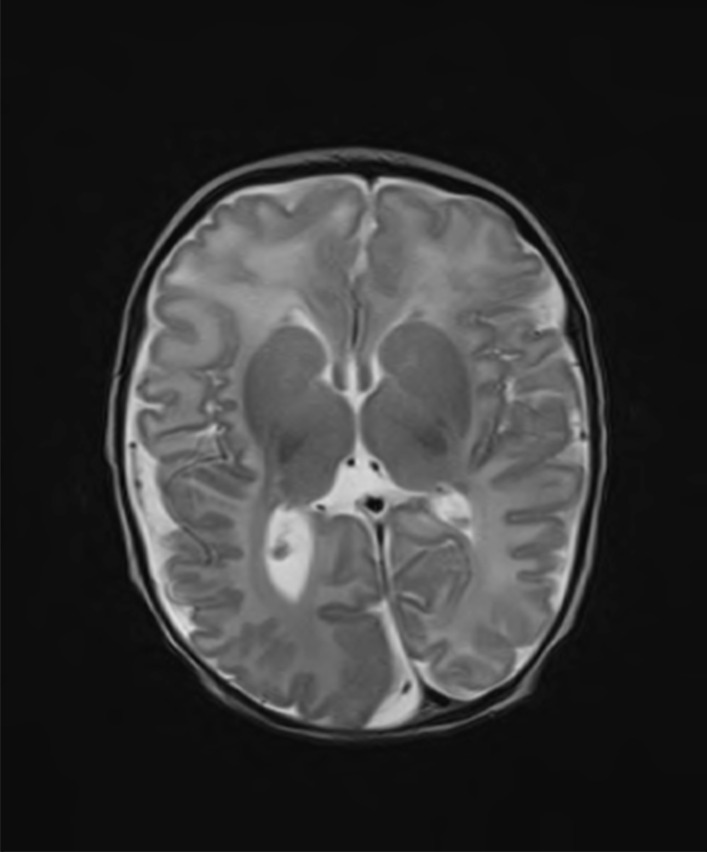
MRI at 2 weeks of age showing right hemimegalencephaly and polymicrogyria of the parietal lobe (patient two)
